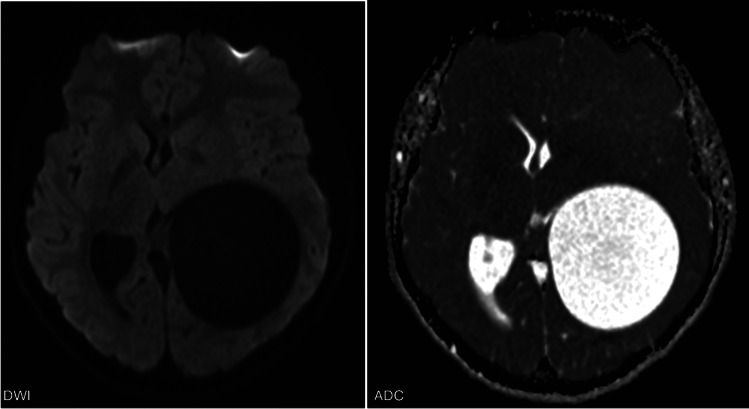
DWI images and ADC map show no diffusion restriction.
